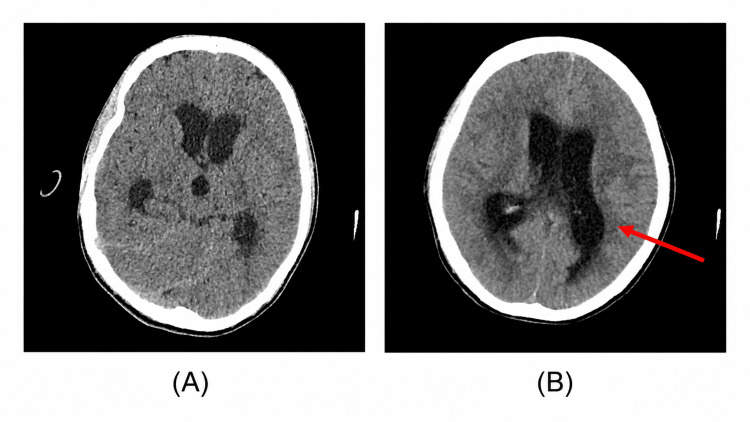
Repeat axial non-contrast CT head images demonstrating new-onset hydrocephalus. (A) Image at the level of the third ventricle showing dilatation of the lateral and third ventricles. (B) Higher axial image showing marked dilatation of the lateral ventricles. No definite focal obstructing lesion was identified on the selected images
